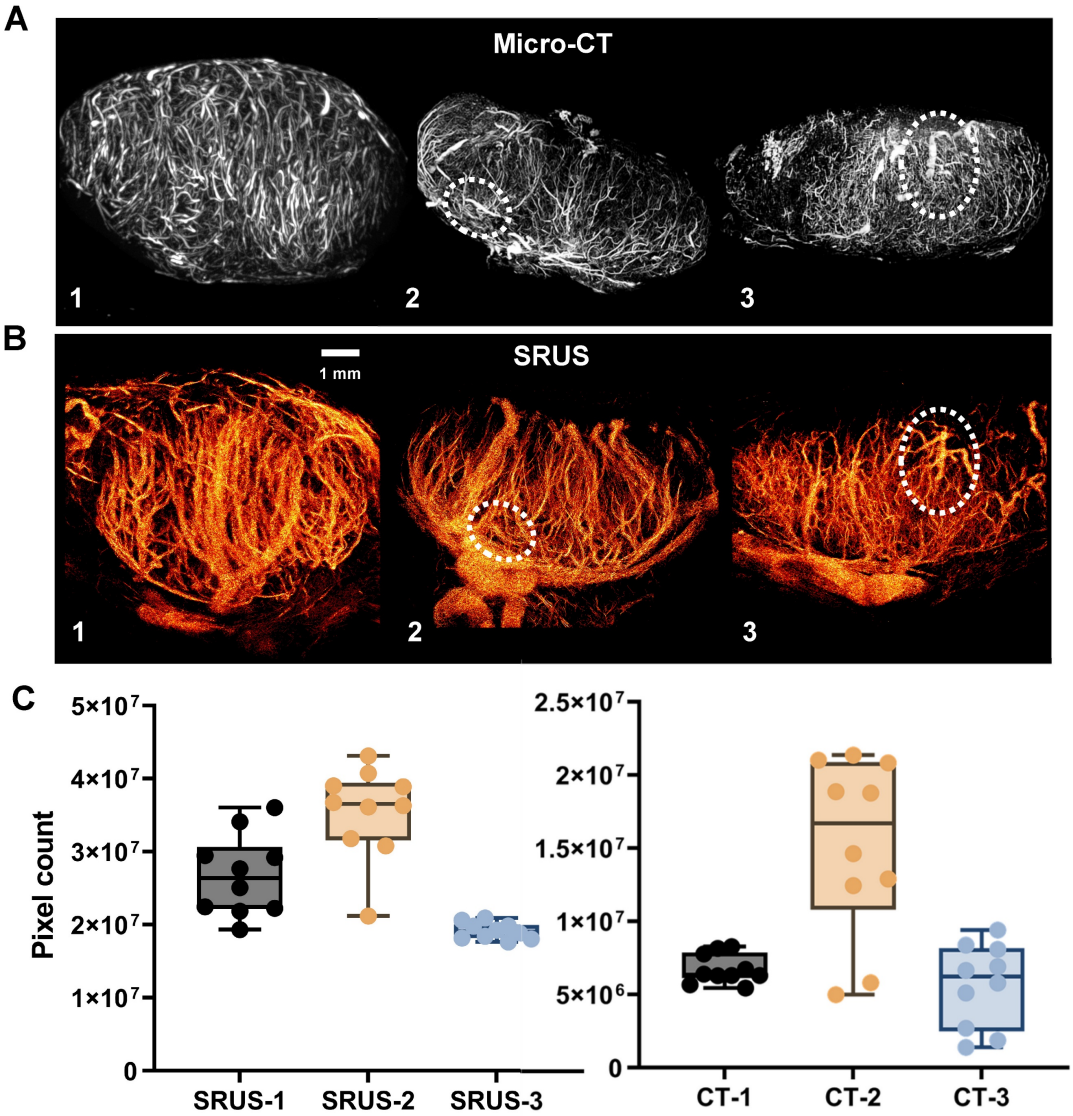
Comparison of SRUS and micro-CT vascular reconstruction results. (A) Representative summed intensity projections of *ex vivo* micro-CT images showing vascular architecture in three lymph nodes (LN 1-3). (B) Corresponding *in vivo* super-resolution ultrasound (SRUS) images acquired from the same lymph nodes. (C) Quantitative comparison of vascular pixel counts between micro-CT and SRUS for each lymph node. Vascular areas were calculated in pixel units from the central 10 imaging planes of each lymph node. Each dot represents an individual image slice. Left panel: SRUS pixel counts across LN 1-3; Right panel: corresponding micro-CT vascular pixel counts. Box plots indicate the median, interquartile range, and full data range. Statistical significance was evaluated using one-way ANOVA.
